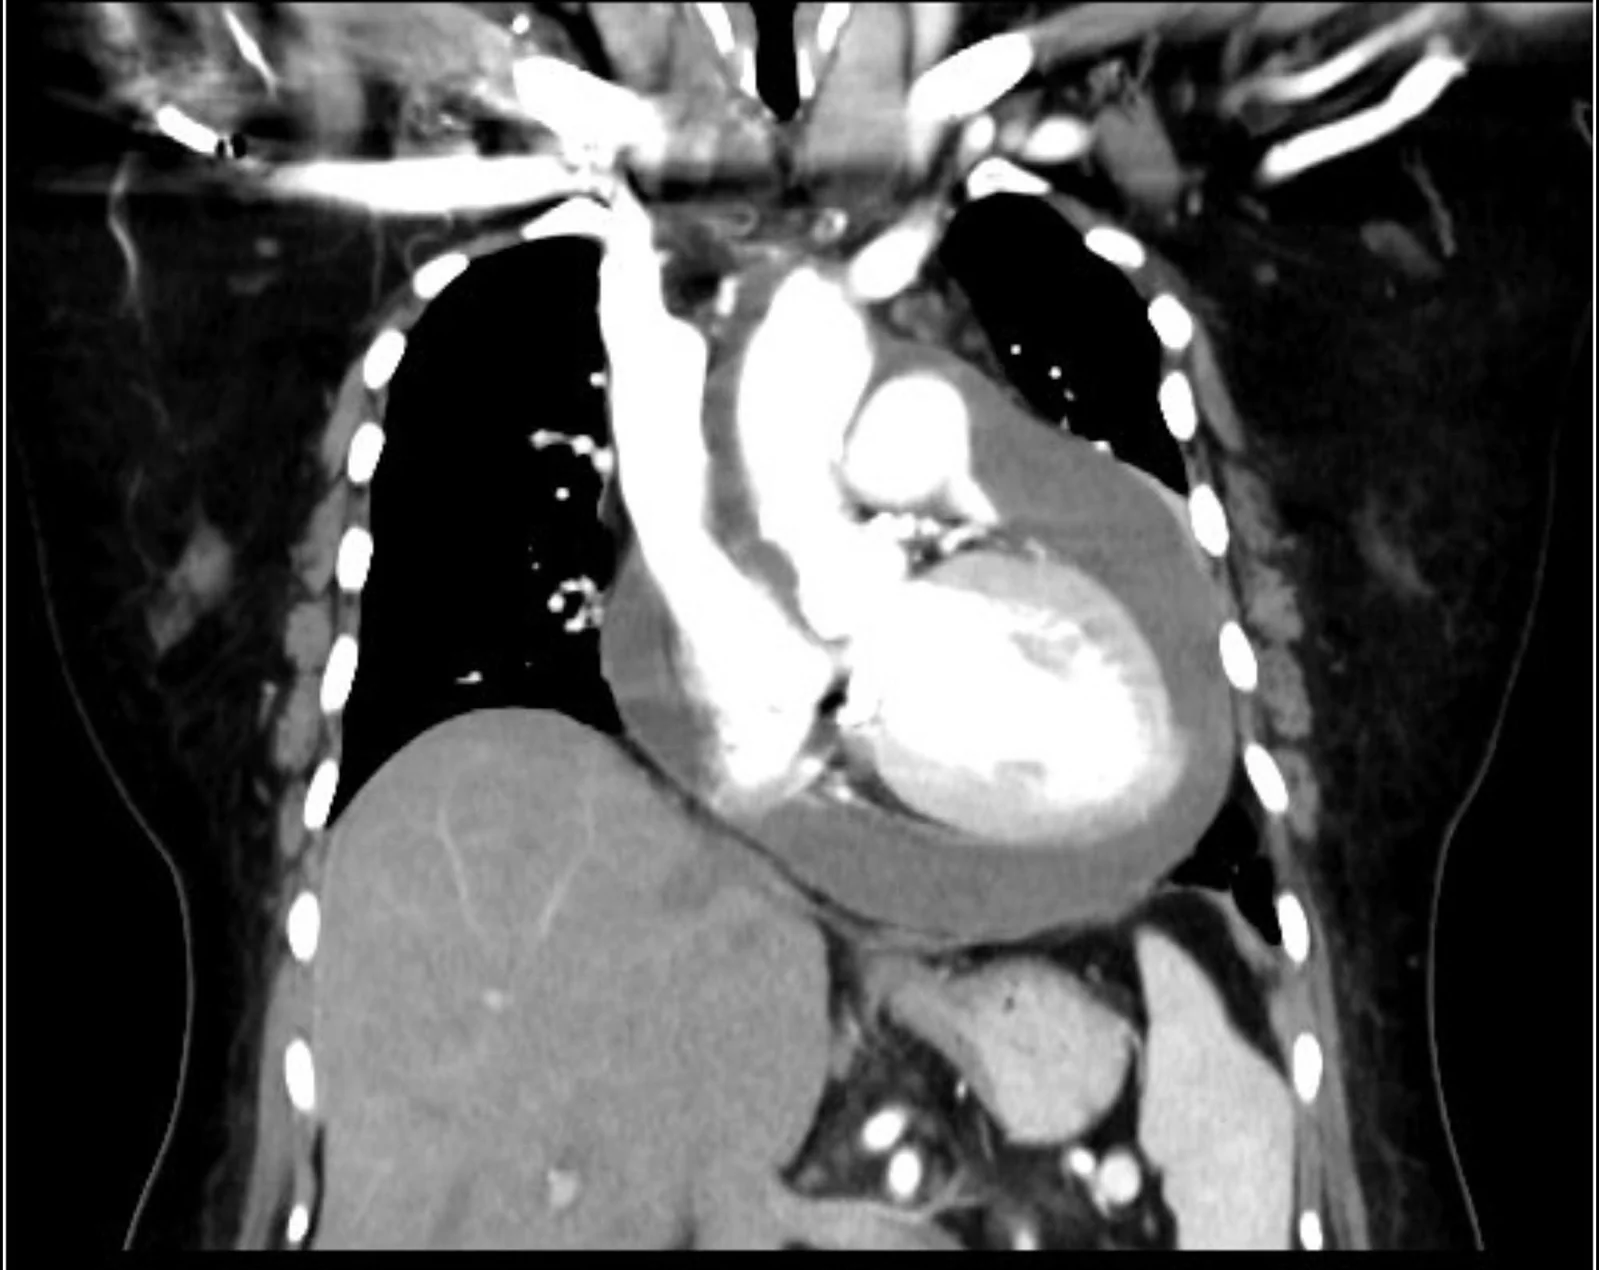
Computed tomography of the chest revealing a massive pericardial effusion.
